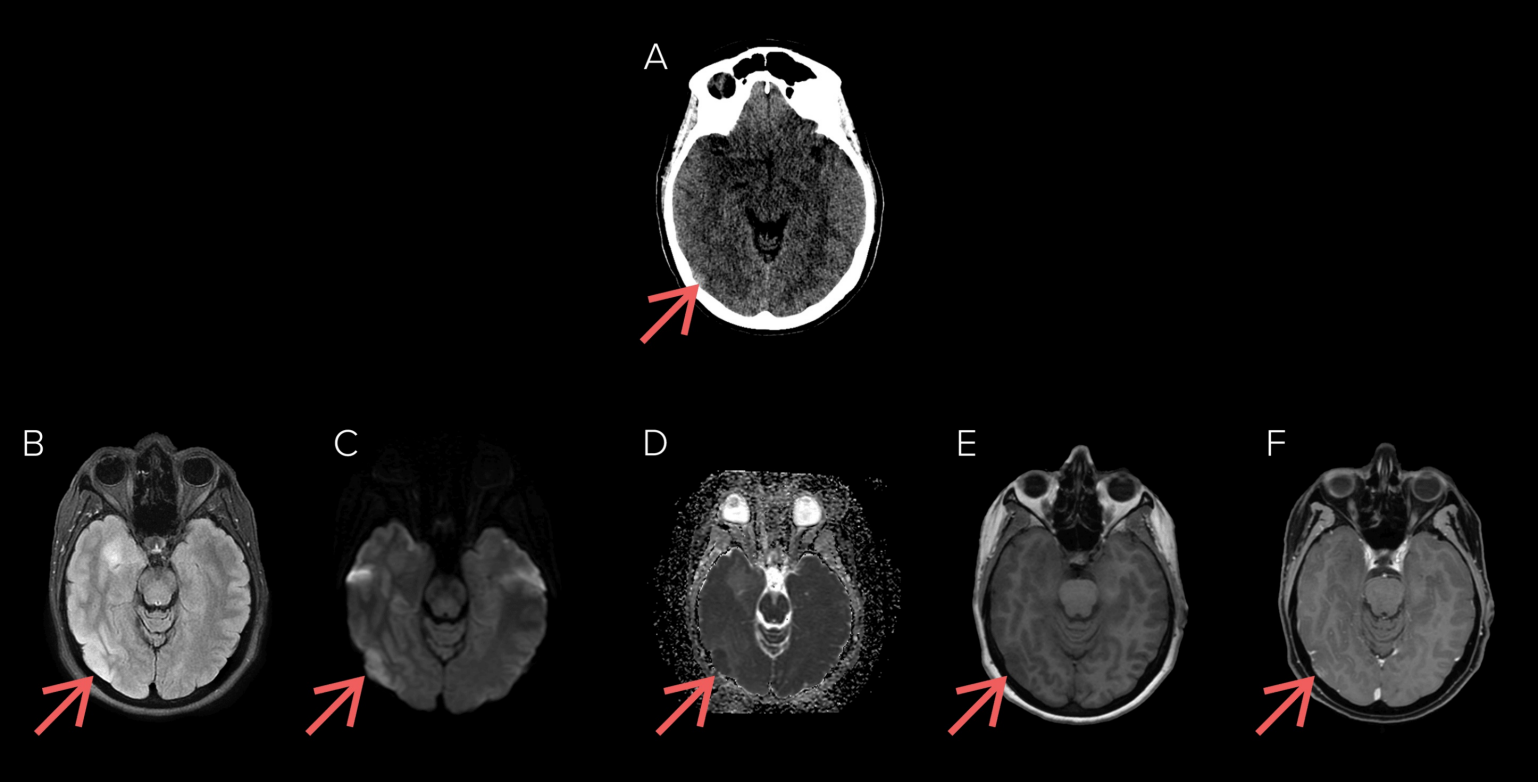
Computed tomography and gadolinium enhanced magnetic resonance images of the brain in a patient presenting with right-sided headache and visual disturbances. (a) Initial non-contrasted computed tomographic images revealed an area of hyperdensity in the right occipital lobe. (b) Fluid-attenuated inversion recovery (FLAIR), (c) diffusion-weighted imaging, (d) apparent diffusion coefficient, (e) pre-contrast T1, and (f) post-contrast T1 sequences revealed a poorly demarcated lesion corresponding to the area of hyperdensity seen on the initial computed tomography study, along with surrounding cerebral oedema. Note, in this case, that there is multicentric disease within the right mesial temporal lobe. Biopsy confirmed glioblastoma.
